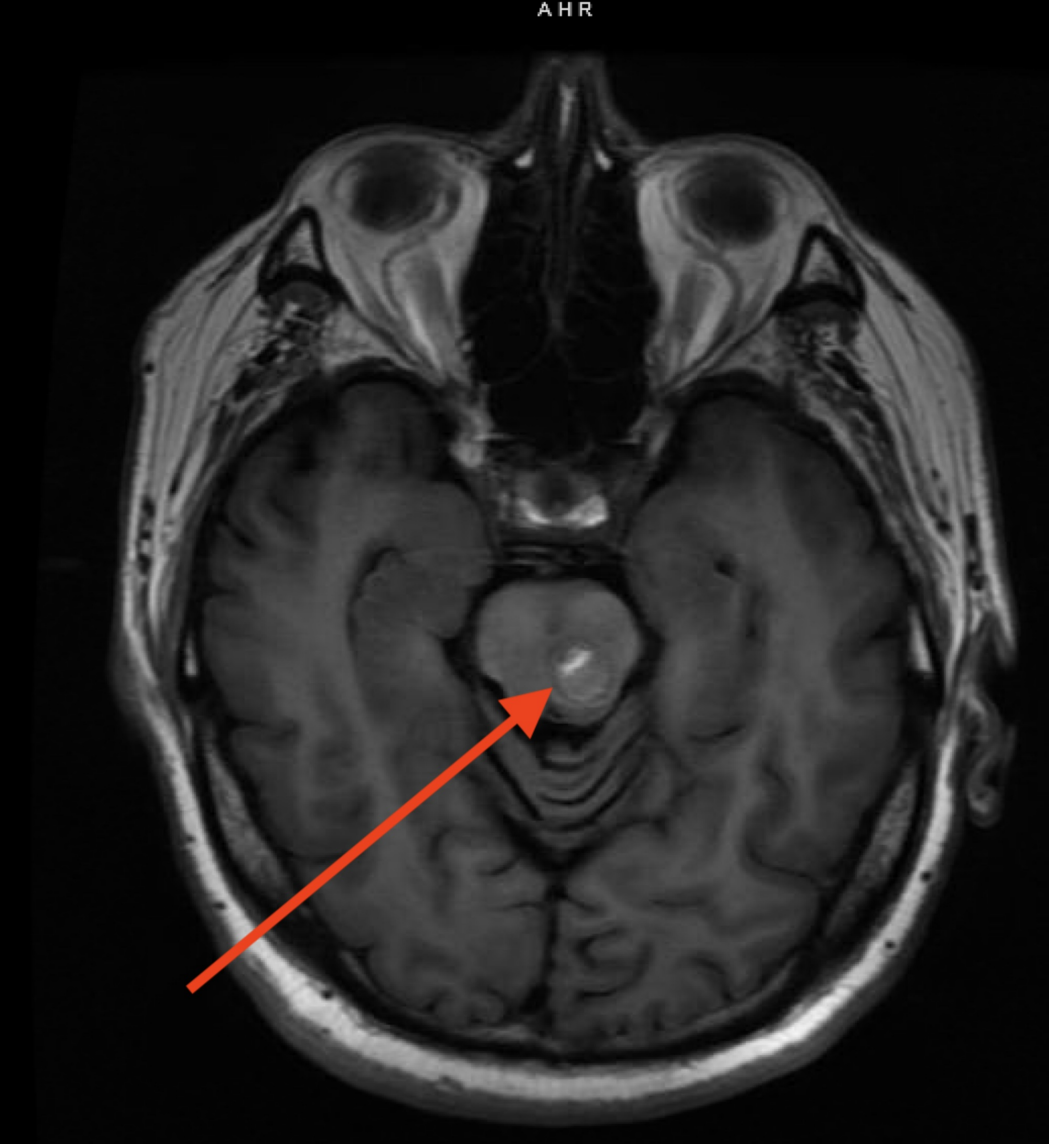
T1 MRI with arrow pointing towards hyperintense signal within angioma cavity.
